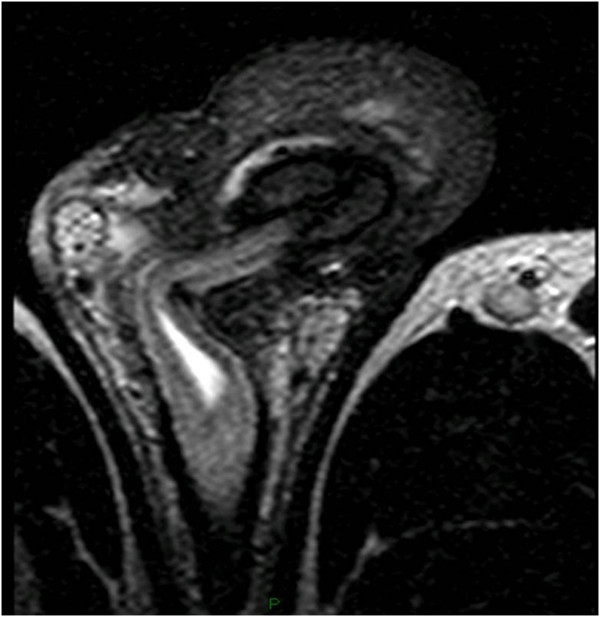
Axial T2-weighted TSA MR imaging showing the fibrotic tissue that leads to a severe narrowing of the penile urethra with dilatation of the upstream tract.
